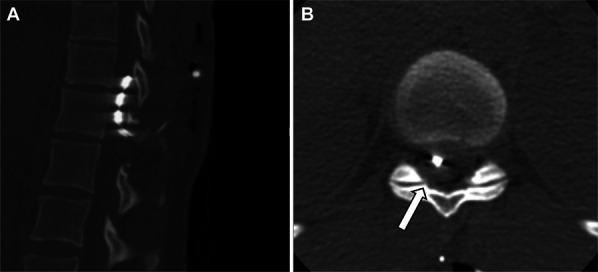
A, and B, CT of the patient presenting with paraparesis after SCS electrode explantation and replacement. B, The arrow is pointing at an epidural hematoma on top of the electrode compressing the spinal cord. This figure is original to this submission, so no credit or license is needed.
